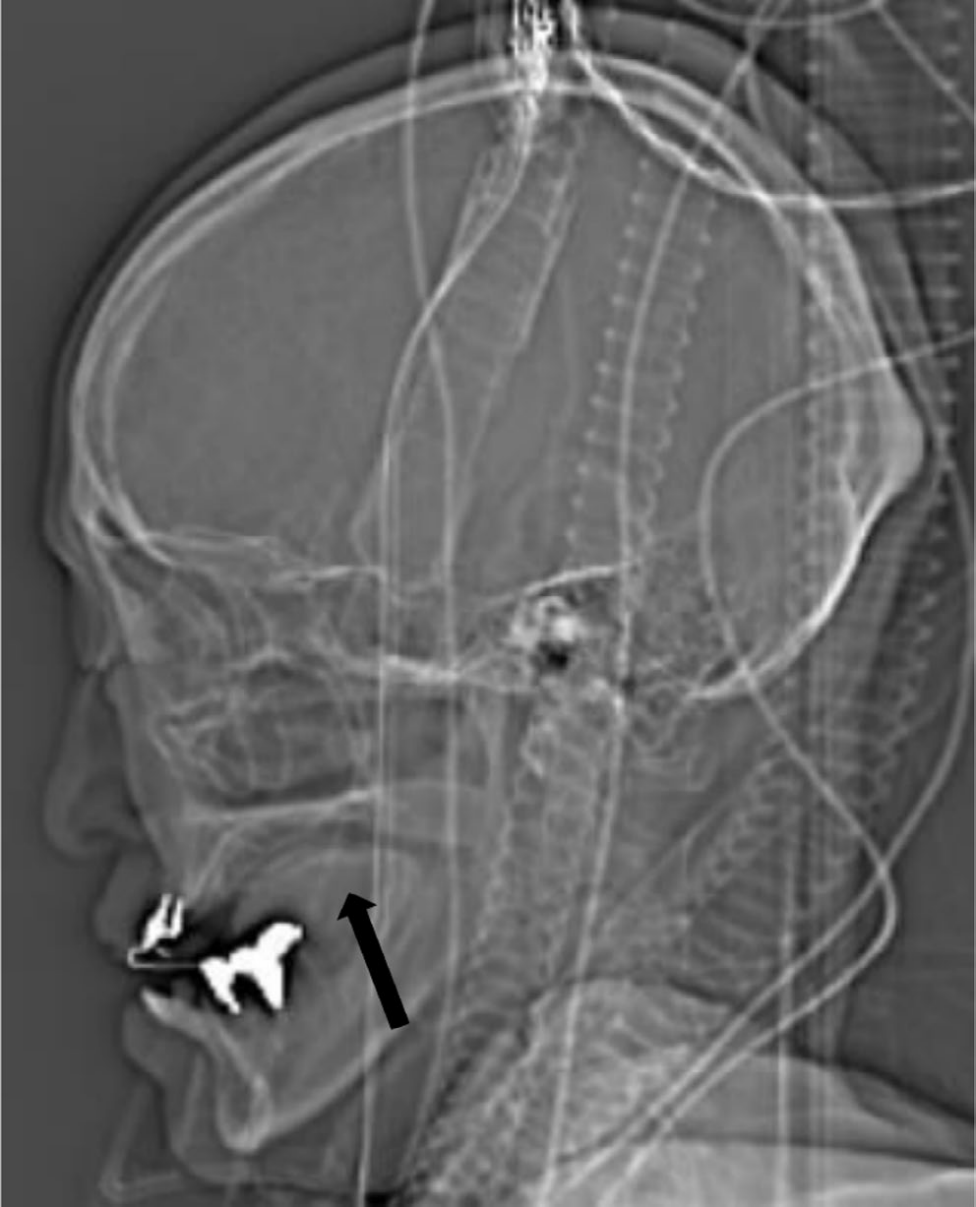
Computed tomography image taken after the onset of subarachnoid hemorrhage at the referring hospital. The tongue (arrow) is stored in the oral cavity.
